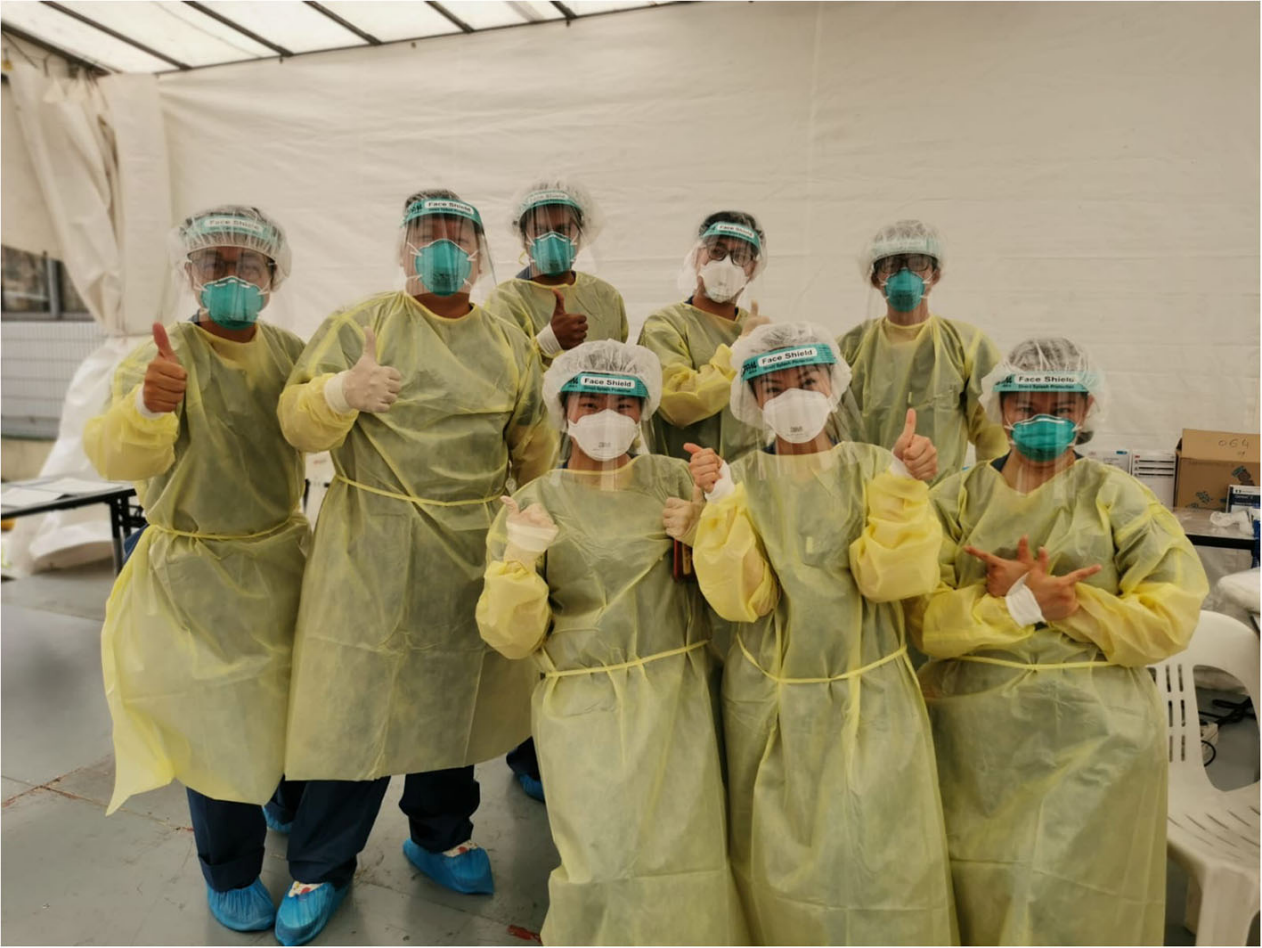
A team of Singapore Health Services (SingHealth) volunteers assigned to manage a medical clinic at a foreign workers' dormitory in Singapore. This team included a neurologist, a dental scientist, a pharmacologist, a cancer patient service associate, a radiographer and nurses (Photo credit: National Dental Centre Singapore).
